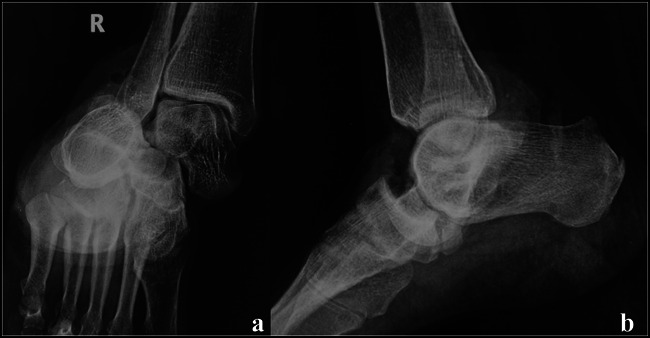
Radiographs of a 45-year-old woman with lateral subtalar dislocation case. A, AP view. (B) Lateral view. Lateral dislocation of the foot under the talus and absence of congruence of the talonavicular and subtalar joints are noted.
